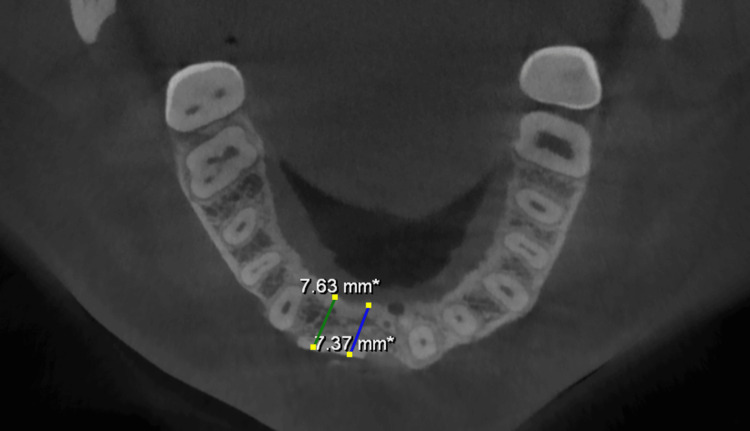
Postoperative CBCT (six months later) - axial view showing a maxillary ridge width of 7.63 mm. CBCT, cone-beam computed tomography
